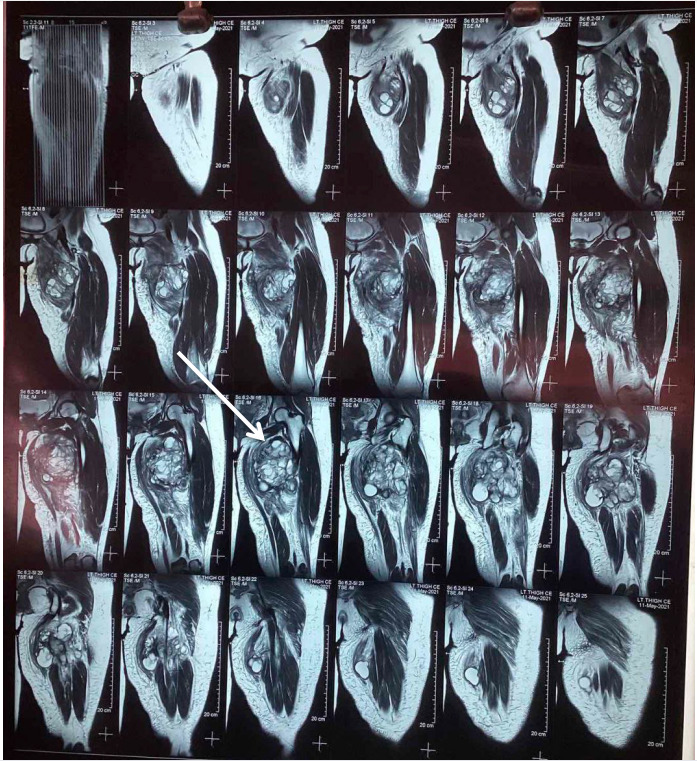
T2 Sequenced MRI showing Triple sign positive.
